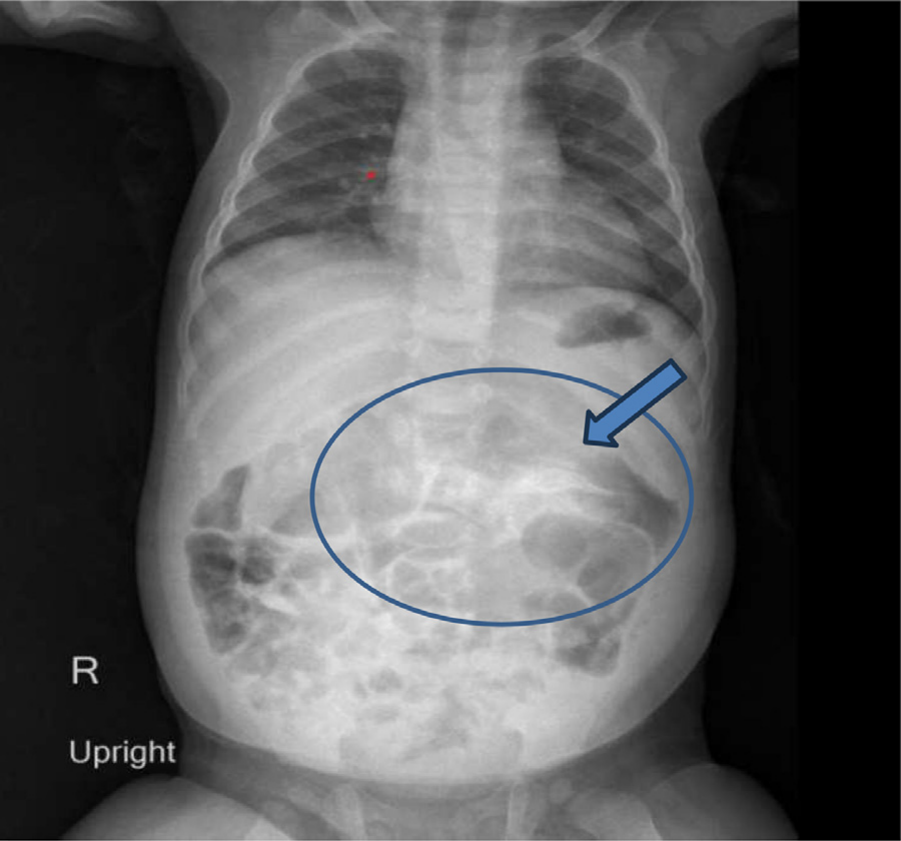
AP Chest and abdominal X-ray* shows curvilinear bony structure (arrow) in the mid abdomen crossing the midline, resembling roughly as axial skeleton. No evidence of abnormal dilatation of bowel loops.
